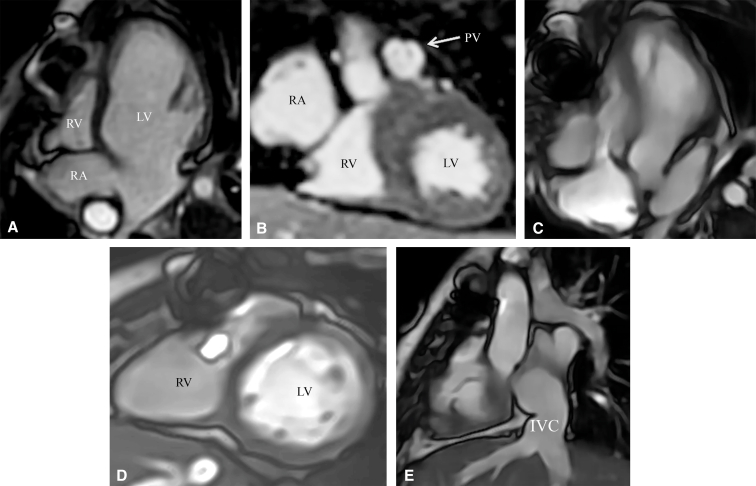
Preoperative and 6-month postoperative cardiac magnetic resonance imaging. A and B, Preoperative studies in 4-chamber and cross-section views, respectively, showing a small right ventricle (RV) beneath the Starnes patch with an RV end-diastolic volume (RVEDV) of 25 mL/m^2^, right ventricle ejection fraction (RVEF) of 10%, and a trileaflet pulmonary valve. C and D, Postoperative studies in 4-chamber and cross-section views, respectively, showing RVEDV of 56 mL/m^2^ and RVEF of 36%. E, Shows the IVC-RA connection. *LV*, Left ventricle; *PV*, pulmonary valve; *IVC*, inferior vena cava.
